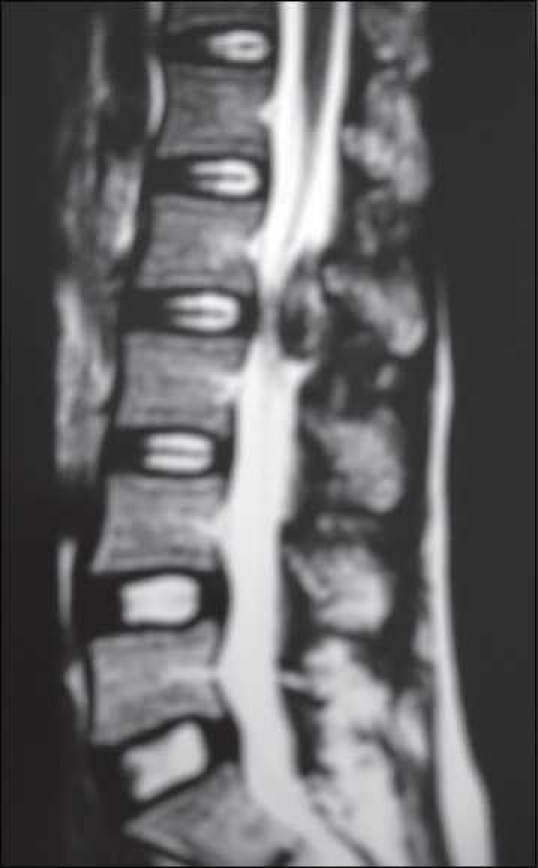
Magnetic resonance imaging picture showing the haematoma compressing the spinal canal
